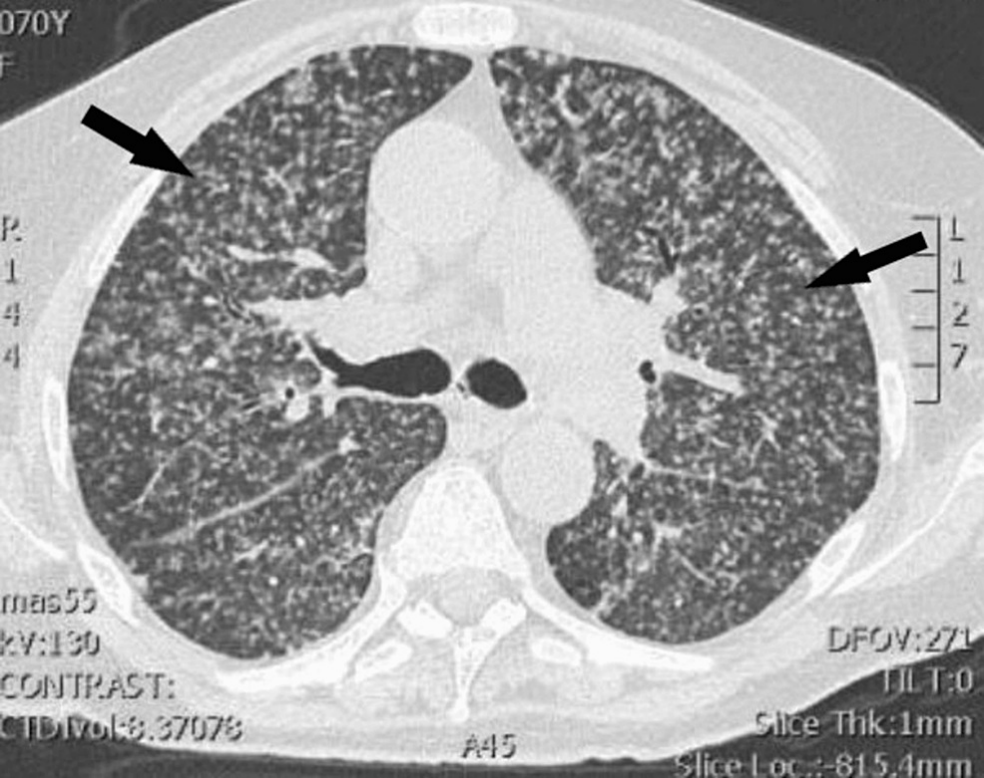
Chest computed tomography without contrast (lung window) Arrows show diffuse bilateral and widespread miliary nodules.
